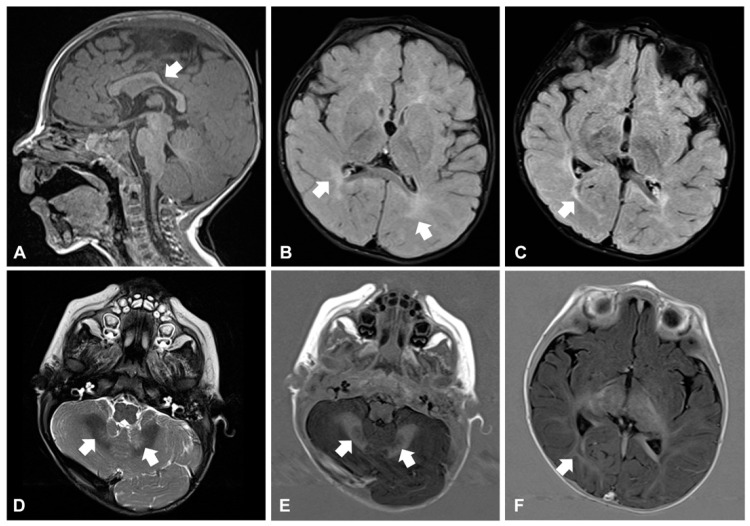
Brain magnetic resonance imaging in patient 1 at age 10 months. Corpus callosum is thin and mildly dysplastic at the level of isthmus (arrow in (A), sagittal view, T1-weighted image). The deep and periventricular white matter appears hyperintense compared to the grey matter (arrows in (B), axial view, FLAIR image), a similar abnormal high signal also involves the optic radiations (arrow in (C), axial view, FLAIR image). Finally, mild hyperintensity of the cerebellar dentate nuclei is visible (arrows in (D), axial view, T2 weighted image). T1-IR images confirm the involvement of dentate nuclei (arrows in (E), axial view) and optic radiations (arrow in (F), axial view).
